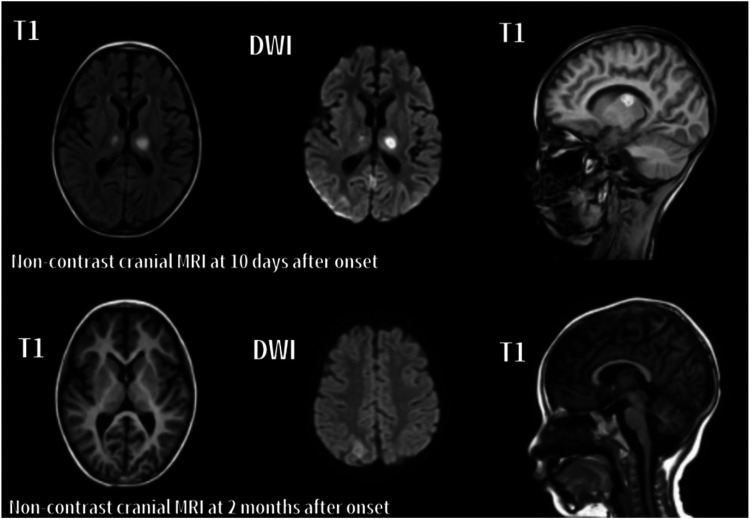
Serial axial and sagittal MRI images of case 7 with ANE. The left and middle columns display axial images, while the right column shows sagittal images. 10 days after onset: Patchy abnormal signals are observed in the bilateral thalami, cerebellar hemispheres, and right parietal lobe. Lesions appear slightly hyperintense on T1, and hyperintense on T2 and DWI. 2 months after onset: T1, T2, and DWI show reduced lesion size and signal intensity in the previously involved regions, indicating radiological resolution.
